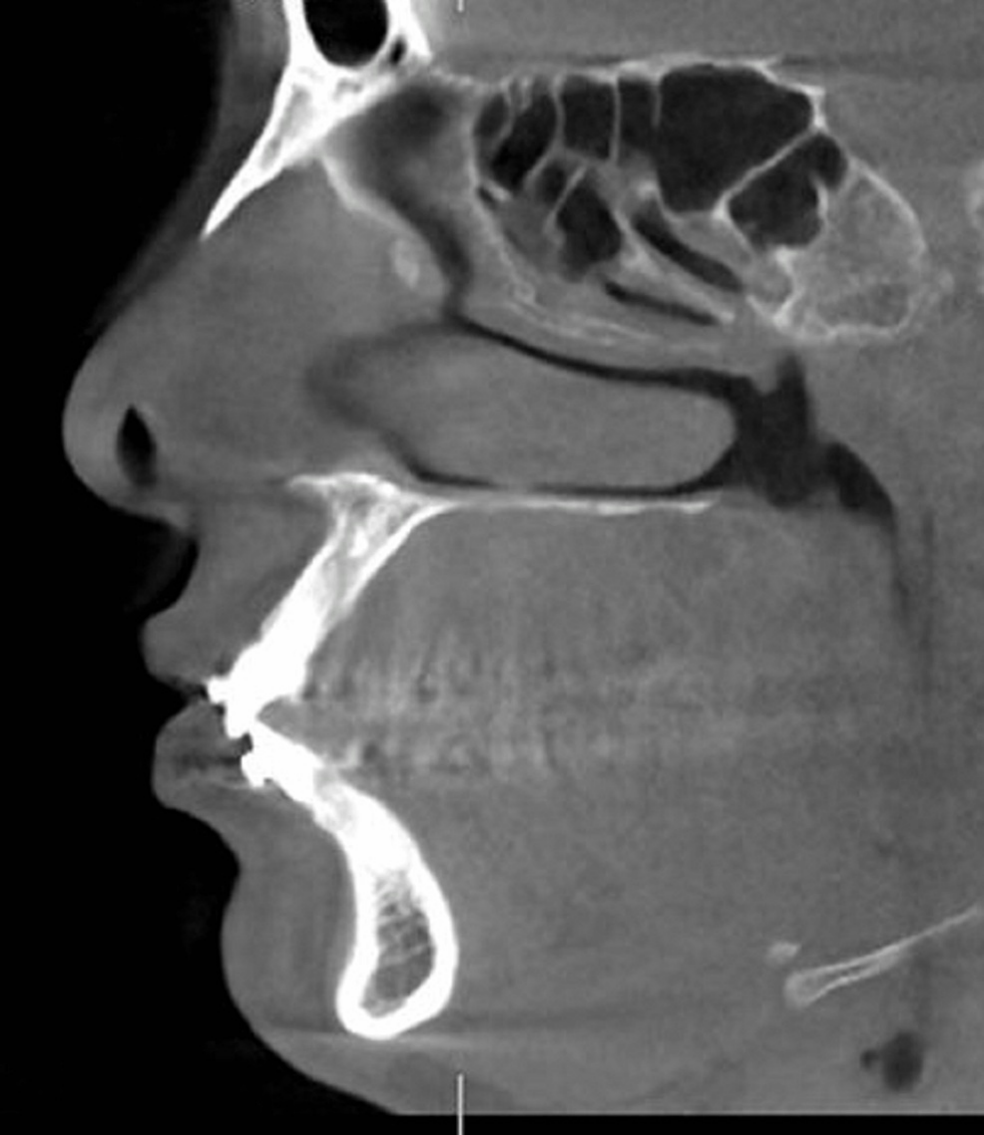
Post treatment with AdvanSync CBCT CBCT - Cone Beam Computed Tomography
